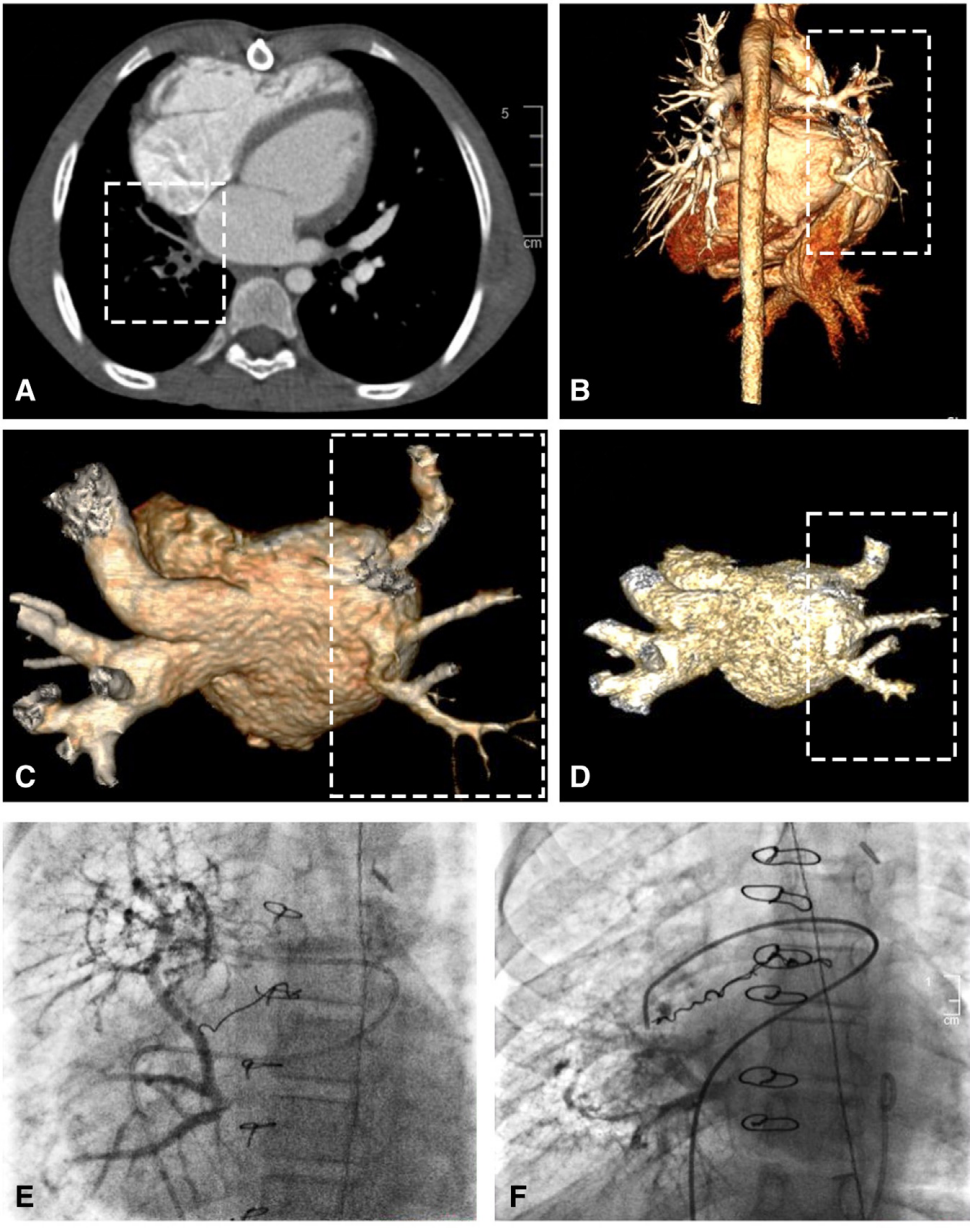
Case 2. A, Preoperative computed tomography scan of the chest demonstrating diminutive RPVs with no connection to the LA. B, Postoperative computed tomography scan of the chest, 3-dimensional reconstruction, demonstrating small-caliber RPVs. C, At 1-year follow-up and D, 2-year follow-up with progressively improving caliber. E, Preoperative angiogram demonstrating occluded RPVs but with distal patency and an extensive network of collaterals and F, postoperative angiography of right pulmonary veins after Kasai-type anastomosis of lung hilum to LA. *RPV*, Right pulmonary vein; *LA*, left atrium.
